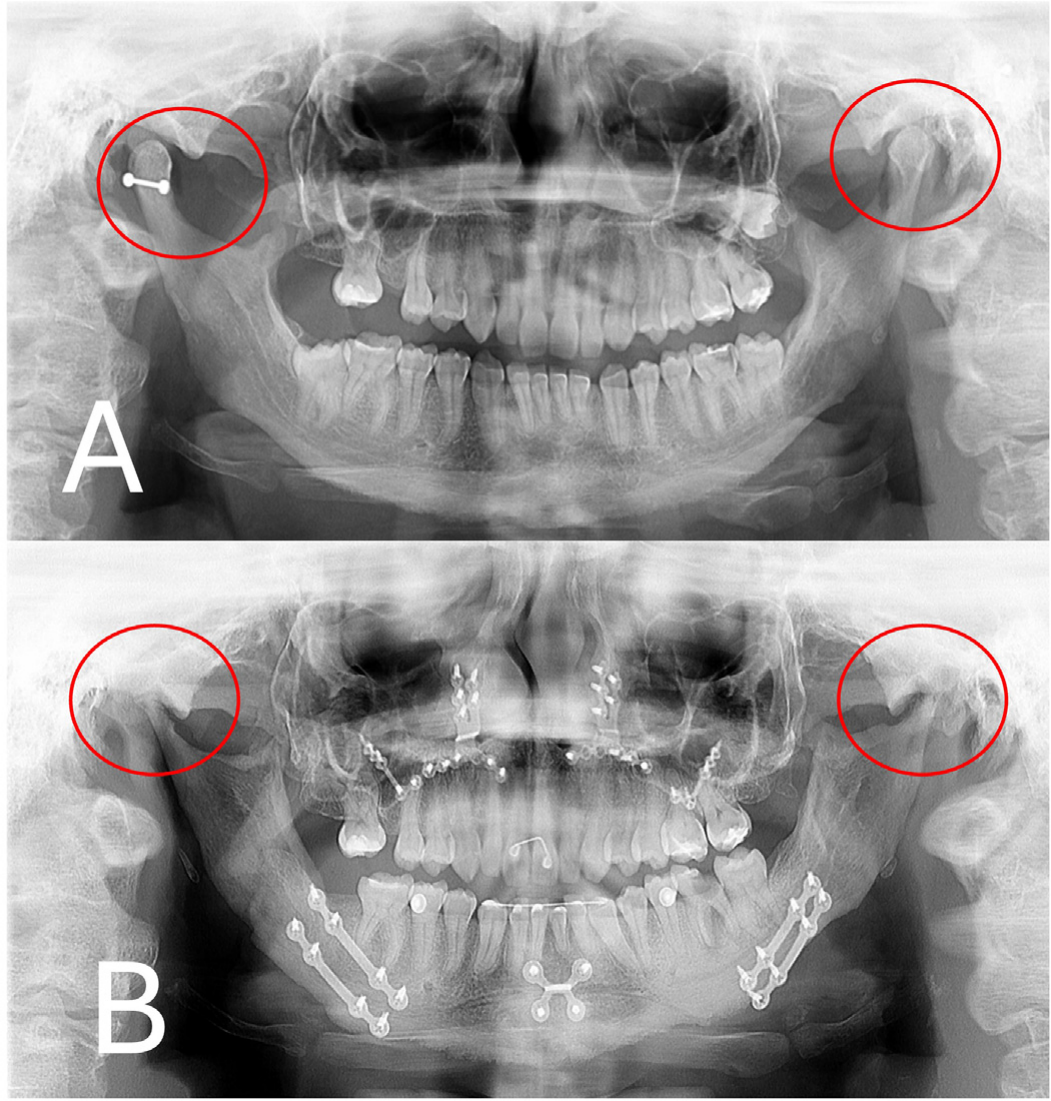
Orthopantomography (A) before orthognathic surgery. Normal condylar process and (B) 2 years after the surgical procedure with condylar process thin and resorbed.
